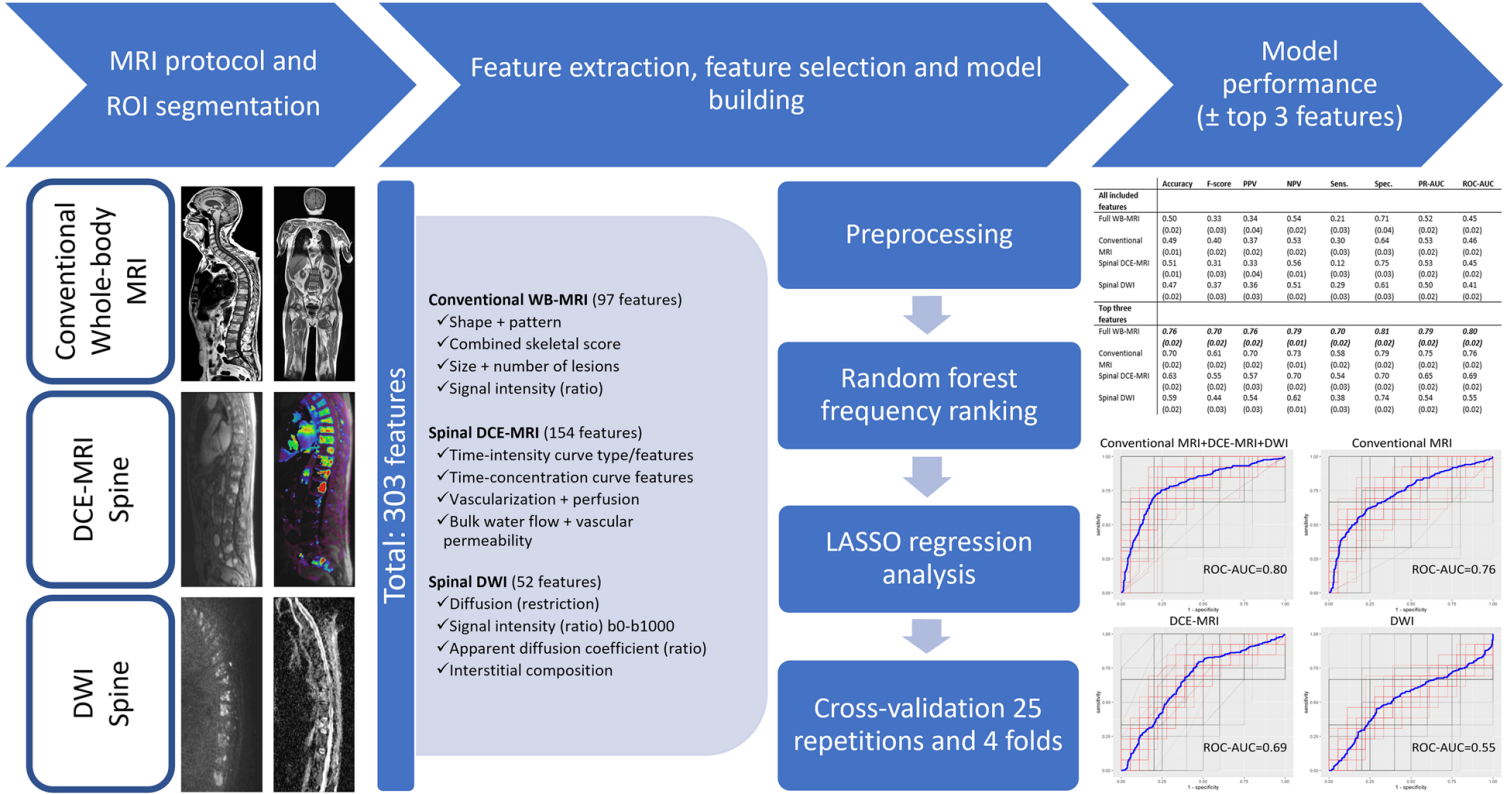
General overview of the MRI protocol and of the methods used for region-of-interest segmentation on the conventional anatomical whole-body MRI, spinal dynamic contrast-enhanced MRI, and spinal diffusion-weighted MRI sequences, for feature extraction, for feature selection, for statistical model building and for testing the models’ performances. In general, models are tested using receiver operating characteristic curve analysis including all MRI features and separate models are retested on the dataset using only the top three most predictive MRI features (in the final model with the three most prevalent features, generalizability can be reduced due to lack of external testing). *AUC* area-under-the-curve, *b0-b1000* diffusion sensitizing gradients, *DCE* dynamic contrast-enhanced, *DWI* diffusion-weighted imaging, *LASSO* least absolute shrinkage and selection operator, *MRI* magnetic resonance imaging, *NPV* negative predictive value, *PPV* positive predictive value, *PR-AUC* precision-recall area-under-the-curve, *ROC* receiver operating characteristic, *ROI* region-of-interest, *sens.* sensitivity, *spec.* specificity, *WB* whole-body
